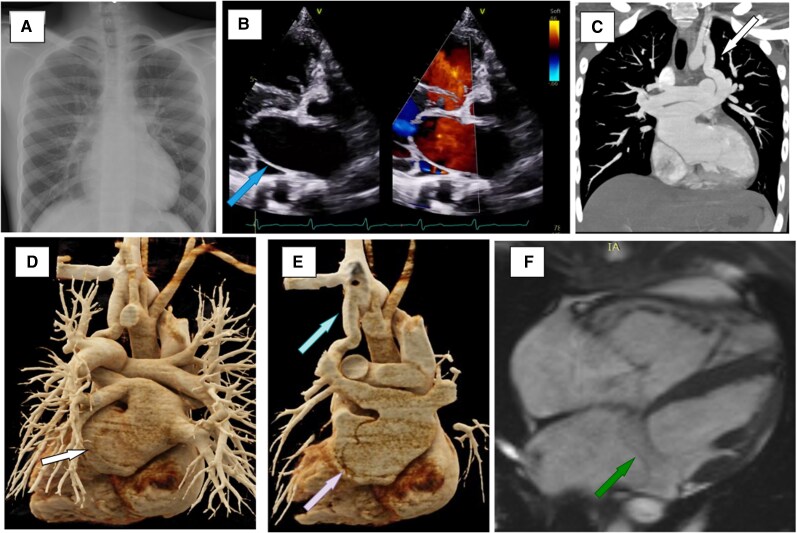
A 22-year-old male patient presented with an acute onset of breathlessness. Chest X-ray postero-anterior (A) view shows mild Grade I pulmonary venous congestion with cephalization in the upper lobe of the lung. Echocardiogram (*B*) shows a dilated left atrium with a thin membrane (blue arrow) dividing the left atrial cavity into two chambers—suggestive of cor-triatriatum. Computed tomography angiogram coronal view (C) demonstrates the levo-atrio-cardinal vein (white arrow) connecting the left upper lobe pulmonary vein to the left innominate vein. The cinematic virtual reconstruction technique images show (D and E) the thin left atrial membrane (white arrow in D and violet arrow in E) along with the levo-atrio-cardinal vein (blue arrow in E). The magnetic resonance imaging 4-chamber view (F) demonstrates the left atrial membrane (green arrow). The patient underwent excision of the membrane with ligation of the levo-atrio-cardinal vein.
